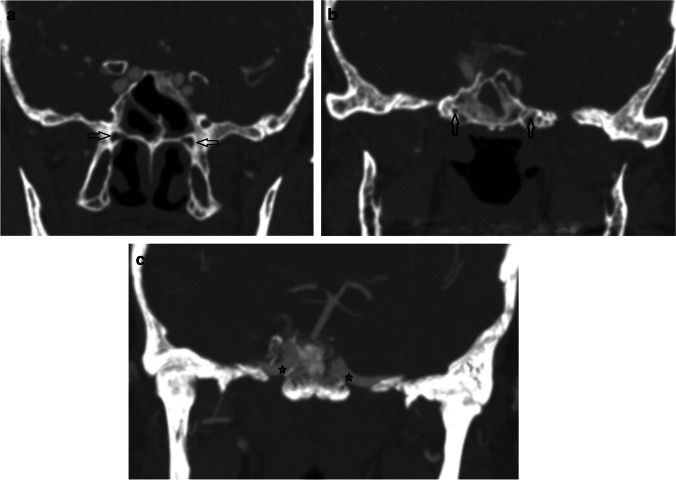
Patient 3. (a) Coronal reformat of the CTA perpendicular to the craniocaudal angle of the VC but without coronal obliquity extending from anteriorly to posteriorly (a–c). The location of the anterior genua of the pICAs (black stars) was recorded as superomedial relative to the VC (black open arrows) on either side
